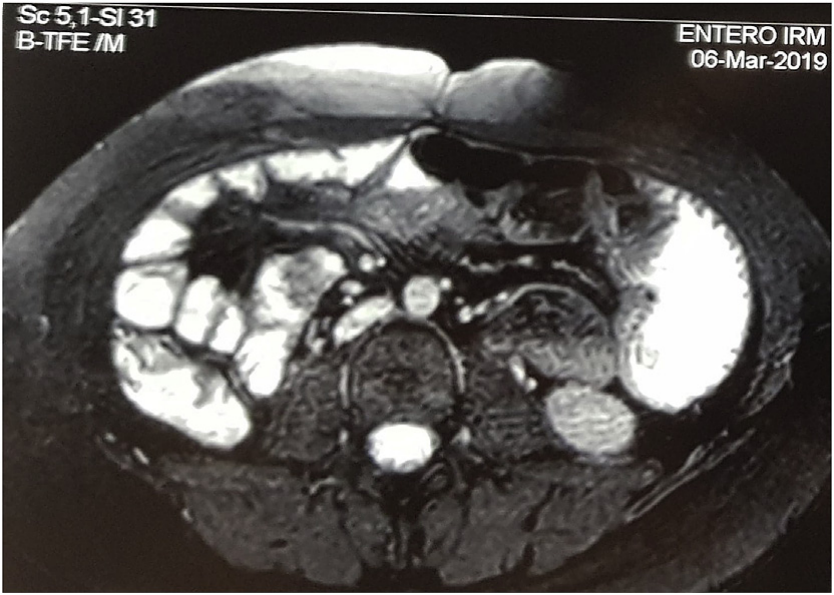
Entero-MRI showing 2 polyps in the right iliac fossa.
